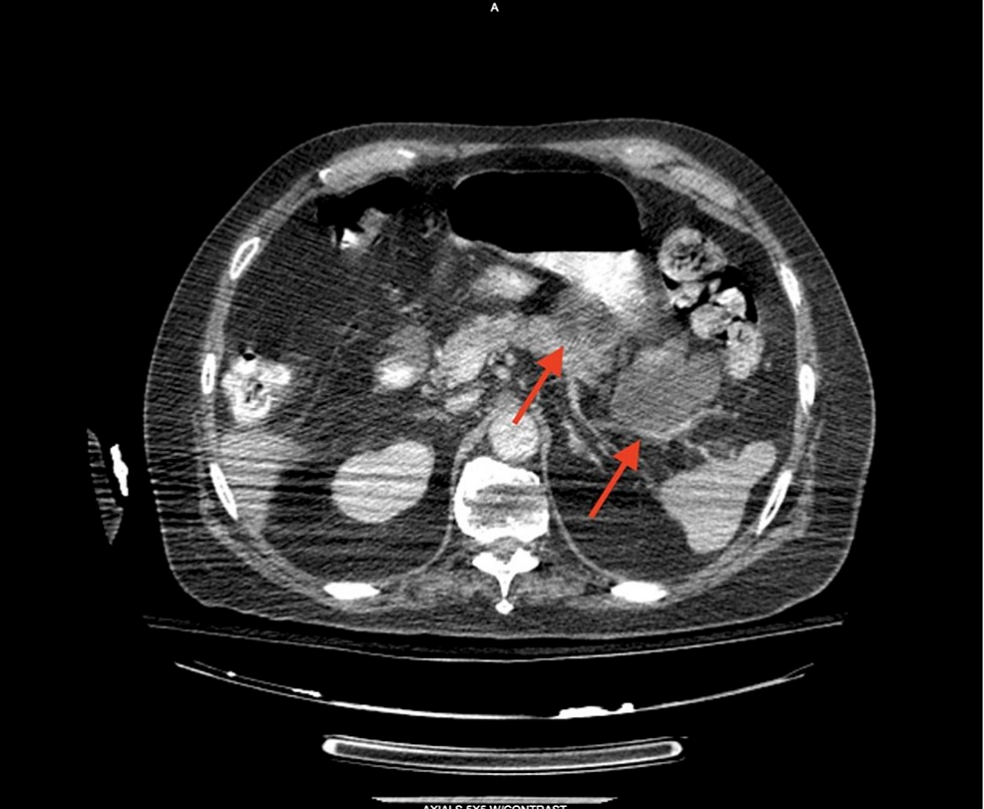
CT with IV contrast of abdomen and pelvis CT with IV contrast of abdomen and pelvis depicting a pancreatic pseudocyst at the tail measuring 5.1x3.9 cm and a pancreatic pseudocyst at the body lesion stable in size, measuring 4.3x2.6 cm (arrows).
